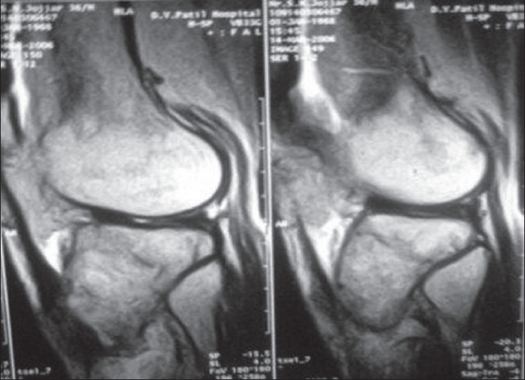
MRI (sagittal section) shows sub articular eccentrically located lesions in the lateral tibial plateau, lateral femoral condyle & patella
